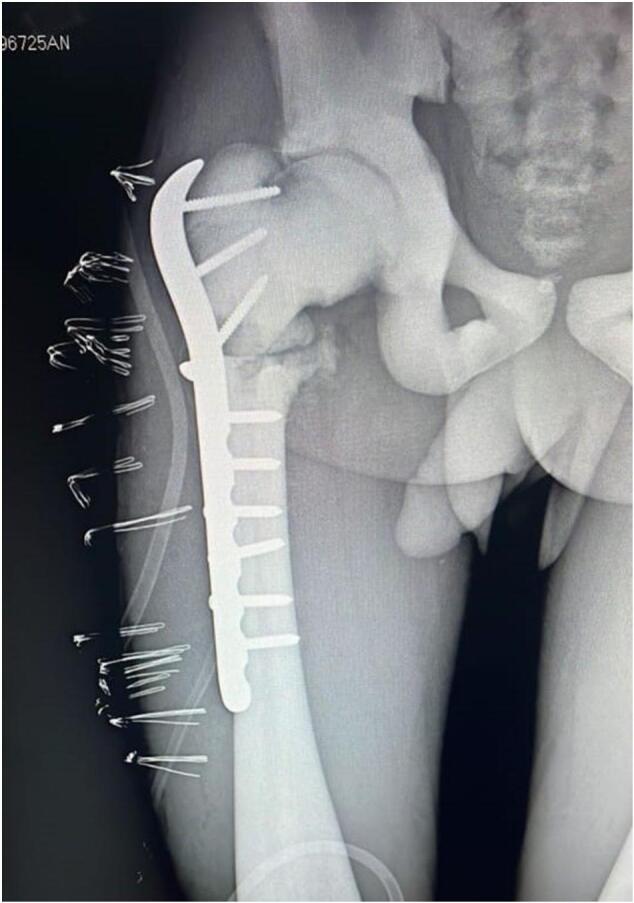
Postoperative X-rays of the left hip.
